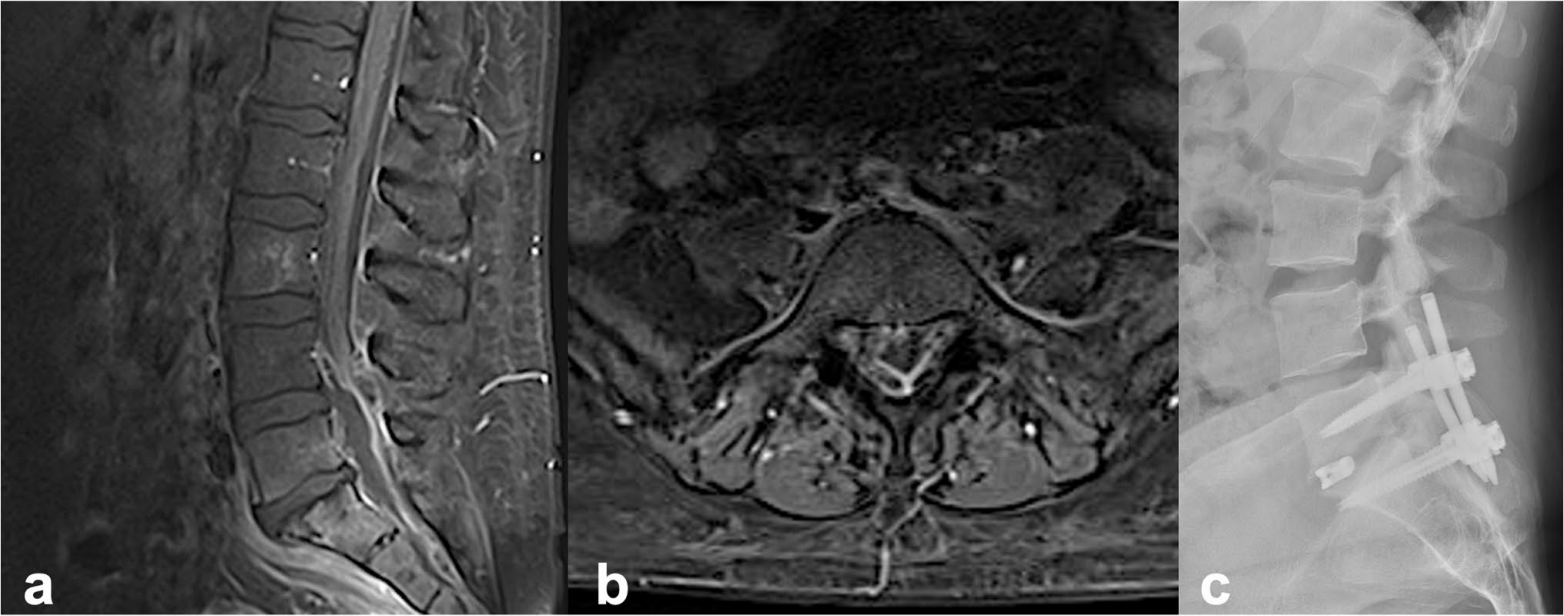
Postcontrast sagittal (a) and axial (b) magnetic resonance (T1 gadolinium sequence) imaging of dorsal lumbar epidural abscess and early end plate destruction of L5 and S1 of an 36-year-old male patient with intravenous drug abuse presenting with lumbar pain and progressive motor weakness of low extremeties. (c) Lateral radiographic view of posterior instrumentation and fusion extending from level L5 to S1
